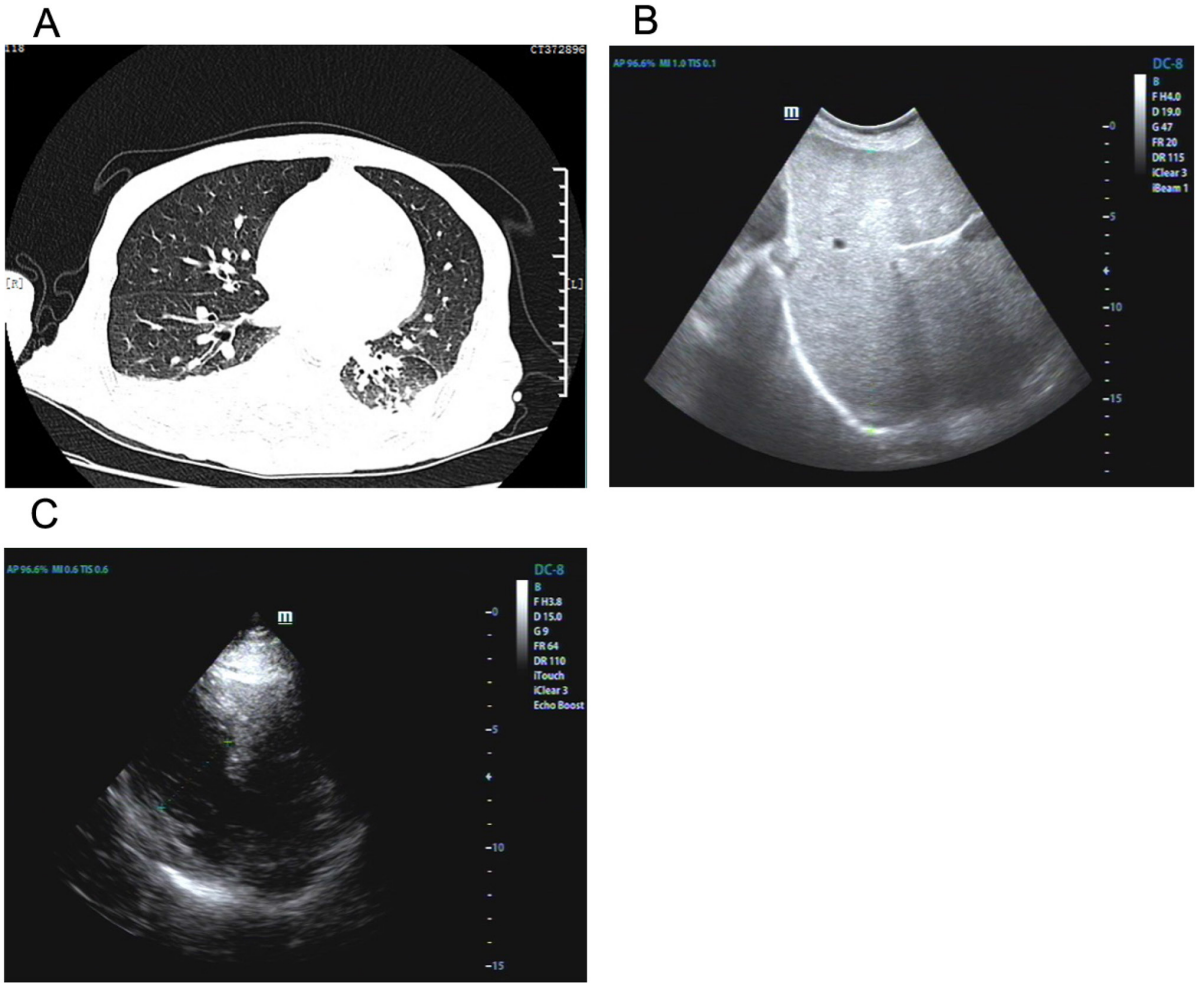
Imaging findings of lung, liver and heart Chest CT showed inflammation and pleural effusion with right basal lobe atelectasis. (A) Abdominal ultrasonography indicated swollen liver (B). Echocardiography demonstrated left ventricular wall thickness was increased (C).
